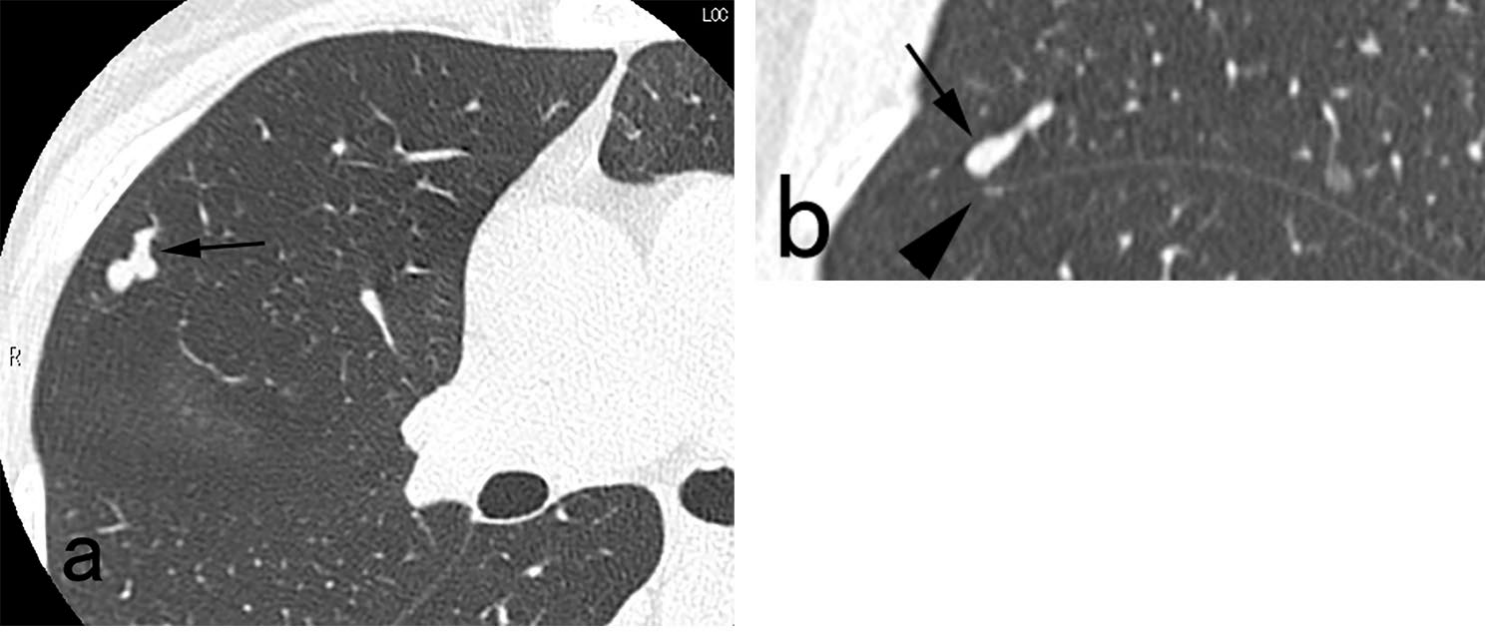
A case with invisible PAVM without pleural bulge. The distance between pleural surface and PAVM (arrow, a) was 4.6 mm. Reformatted sagittal CT image clearly depicted the relationship between the PAVM (arrow, a) and the margin of the incomplete minor fissure (arrowhead, b). The surgeon promptly detected the PAVM soon after making incision into the incomplete minor fissure, and accomplished wedge resection of the PAVM within 24 min
